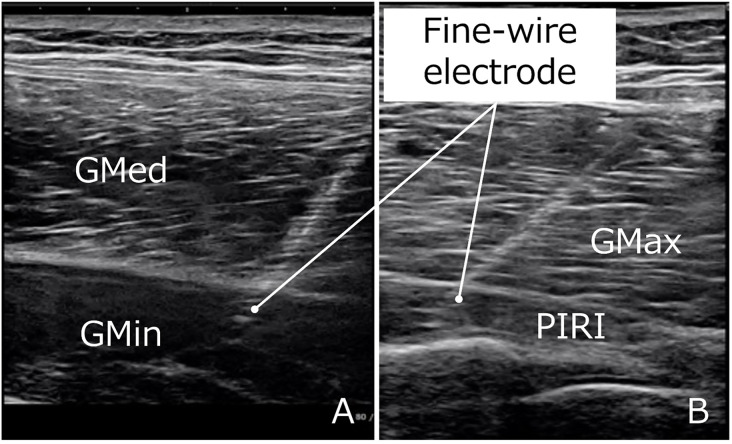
Ultrasound imaging showing insertion of fine-wire electrodes into the (A) GMin and (B) PIRI. GMin, gluteus minimus; PIRI, piriformis.
